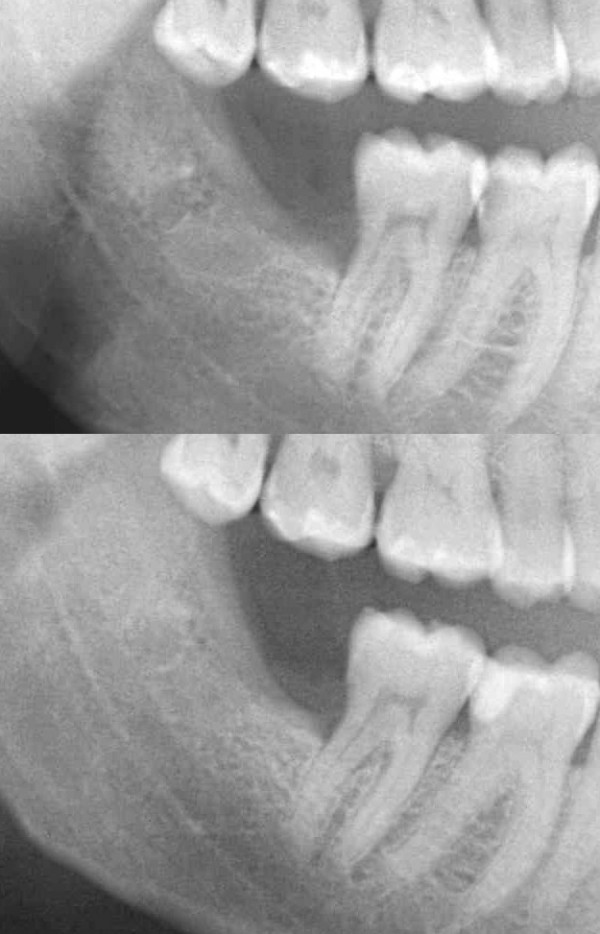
(a) An immediate postoperative dental panoramic tomography was obtained to be considered as a baseline image for this case study. (b) Radiographic image, obtained 50 days postoperatively showing enhancement of radiopacity in the third molar socket area.
